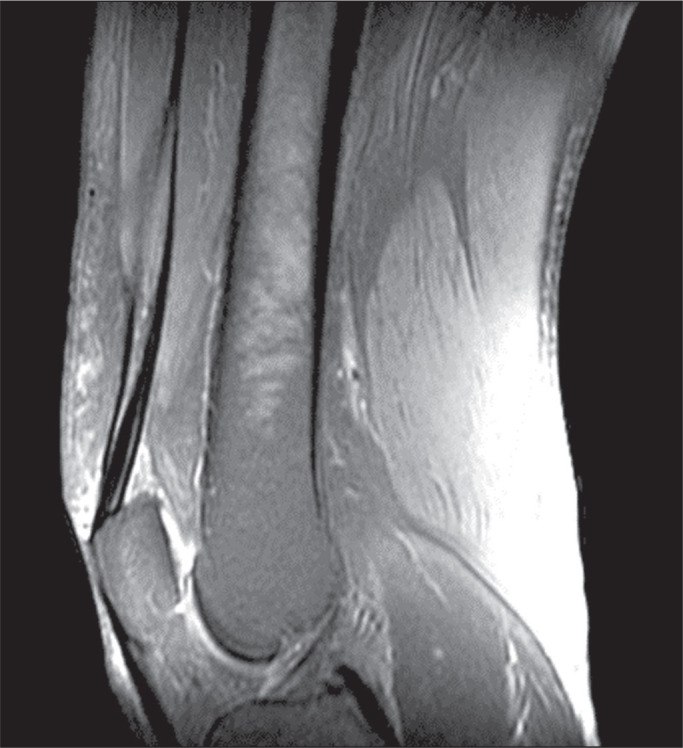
MRI of the knee, showing a diffuse hyperintense signal in the quadriceps
